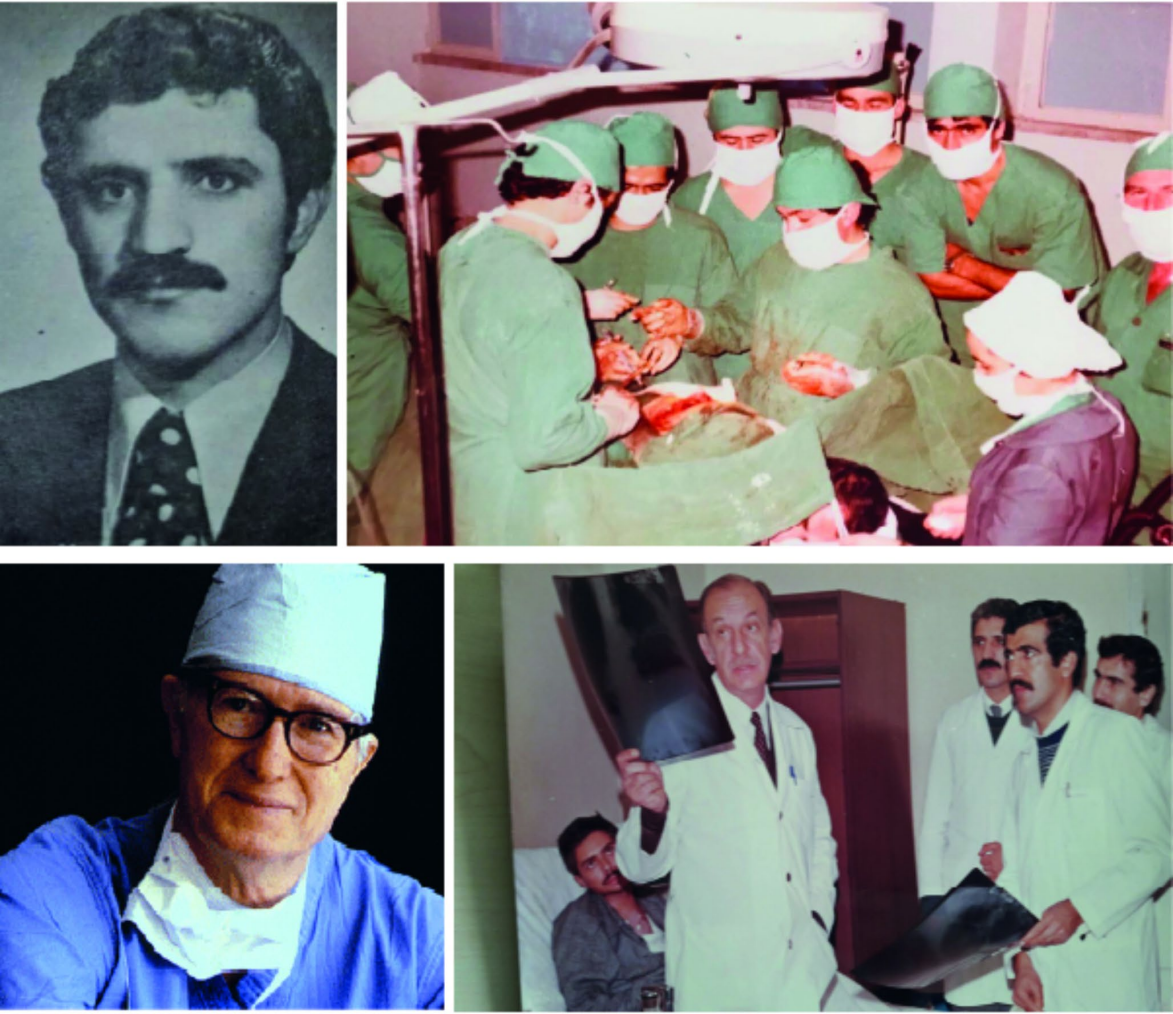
A. Dr. Ismail Demir in his medical school years. B. During a thoracotomy for a lung cancer (left on the image), C. Dr. James Hardy, who performed world’s first human lung transplantation in 1963. D. Prof. Isin and Dr. Demir, and the thoracic surgical team analysing a chest X-ray during a surgical round
